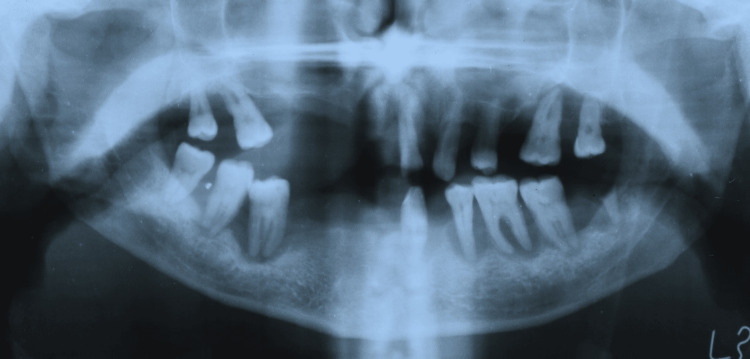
Orthopantomogram showing severe periodontitis and multiple missing teeth
